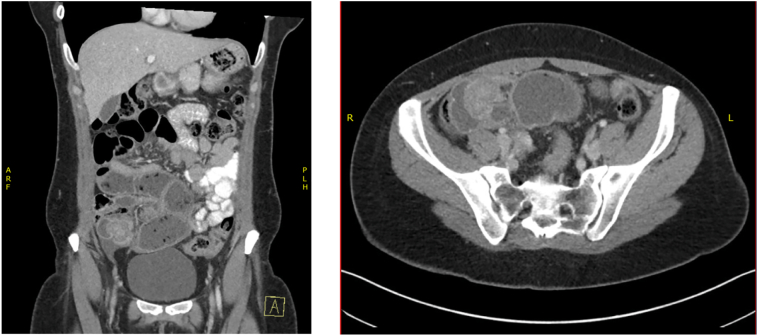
A (coronal view) and B (axial view) of CT abdomen/pelvis demonstrating small bowel dilation and polypoid lesions as highlighted by blue arrows.
